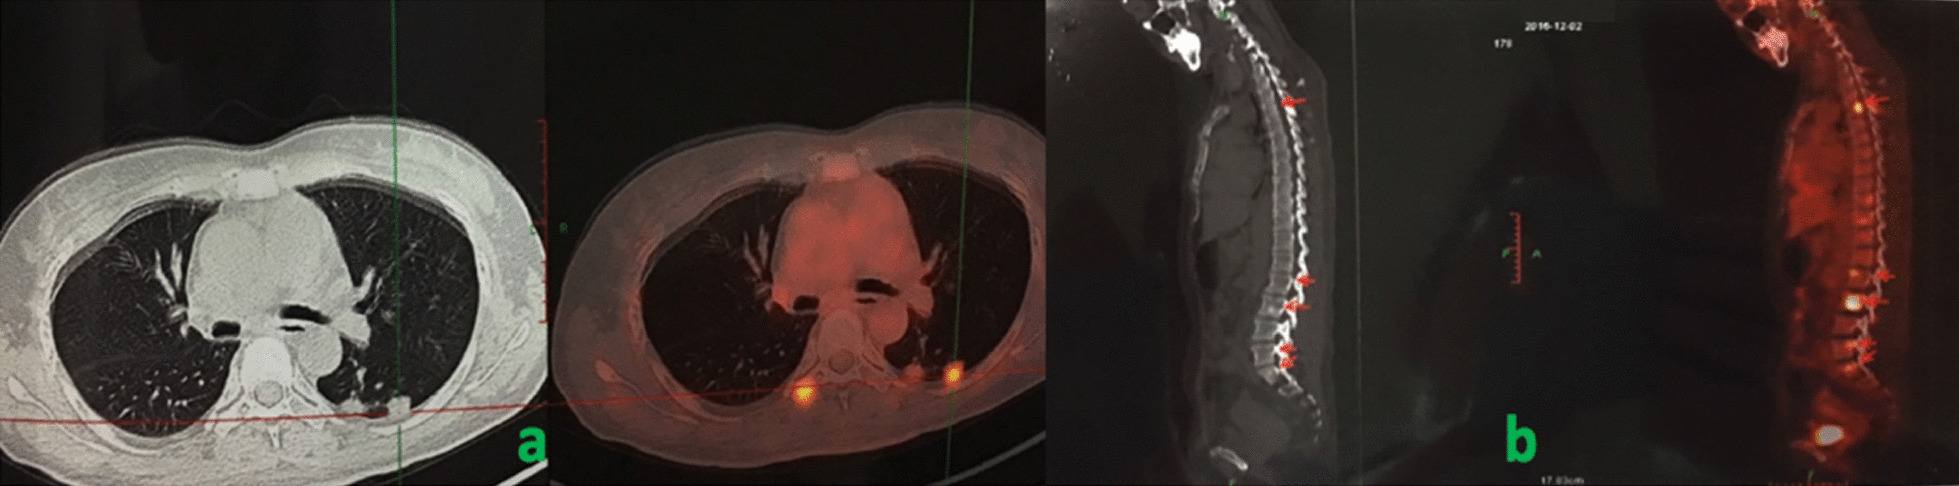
PET/CT showing multiple lesions with high 18F-FDG uptake in lung (a) and vertebral bodies (b)
